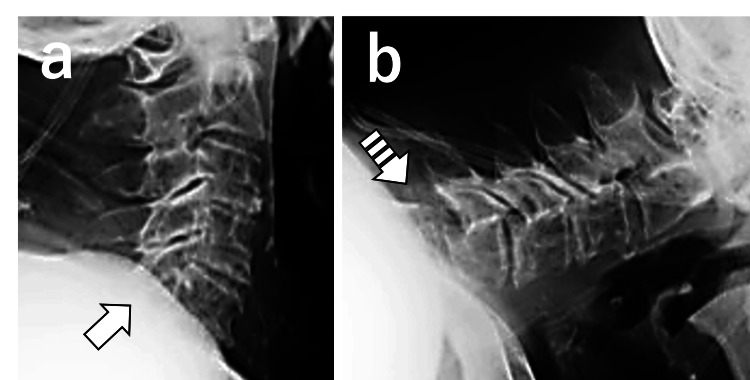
Dynamic lateral cervical spine radiographs taken with a portable X-ray machine. (a) Extension view in which the C5/6 alignment appears reduced. The facet joints are partially obscured by the shoulders. (b) Flexion view showing anterior subluxation at the C5/6 level (striped arrow), consistent with instability.
